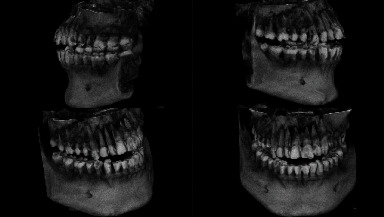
3D CBCT view of case 1 showing resorption over the maxillary alveolus region on the right side.
